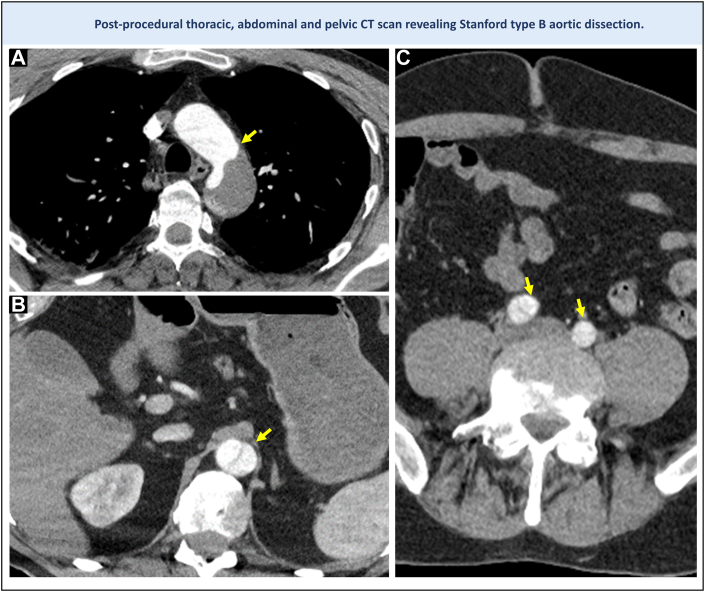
Computed Tomography Revealing a Serious Complication of Endovascular Occluder Recovery (A) Abdominal computed tomography demonstrating a dissection flap (arrow) at the level of the aortic arch, distal to the subclavian artery. (B) Aortic dissection originating at the site of occluder embolization (arrow). (C) Aortic dissection extending distally to both iliac arteries (arrows). PFO = patent foramen ovale.
